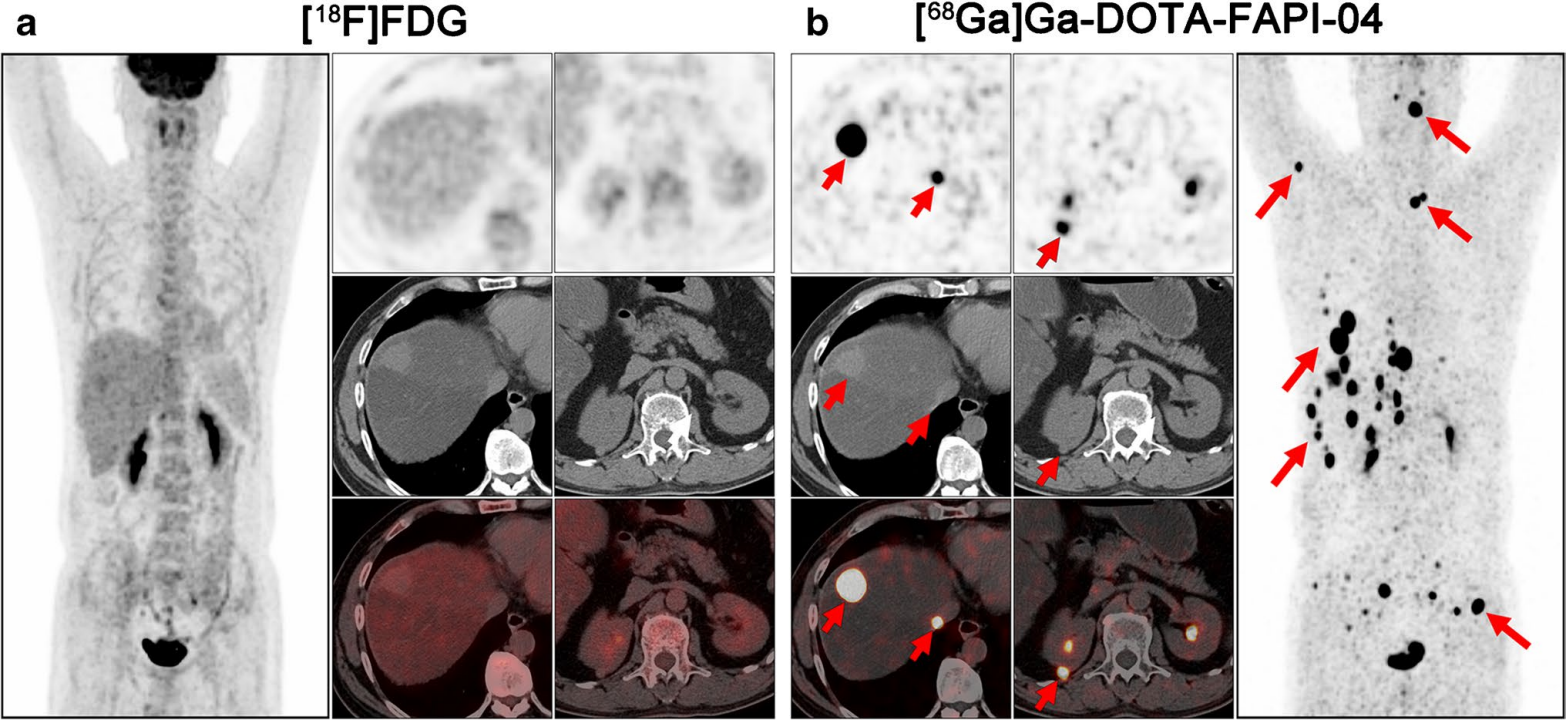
A 48-year-old man (patient #45) pathologically confirmed with liver interdigitating dendritic cell sarcoma (IDCS) received radical operation 2 years ago. [^18^F]FDG PET/CT (a) demonstrated none of the [^18^F]FDG-avid lesions. Interestingly, [^68^ Ga]Ga-DOTA-FAPI-04 PET/CT (b) demonstrated all the metastatic lesions with intense [^68^ Ga]Ga-DOTA-FAPI-04 activity, including liver, right kidney, and bone metastases. Red arrows indicated the tumor lesions detected by [^68^ Ga]Ga-DOTA-FAPI-04 alone
